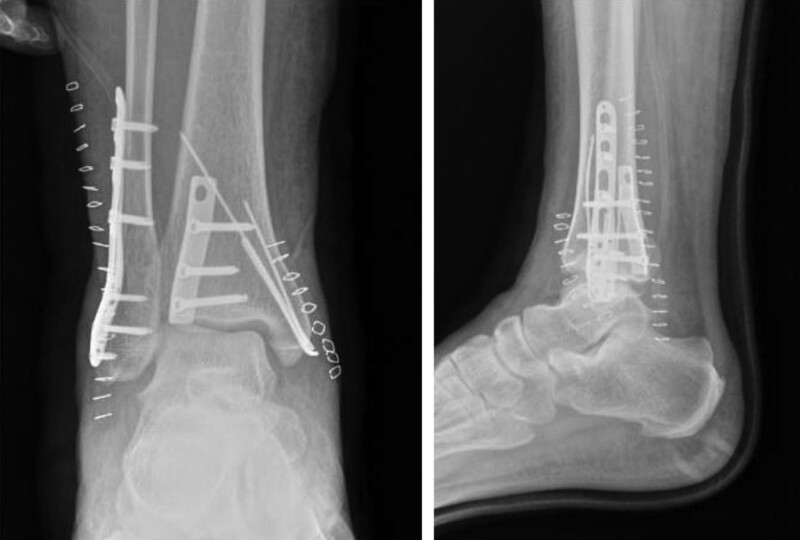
X-ray imaging after surgery. No obvious abnormalities was found.
